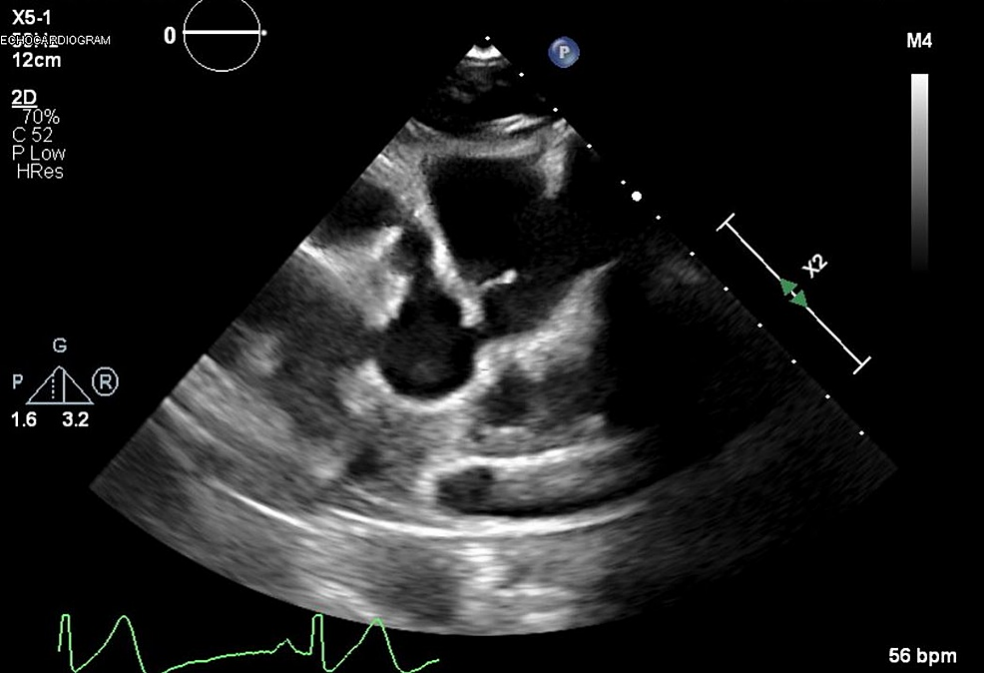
TTE-modified parasternal short-axis view demonstrating giant left and right coronary artery aneurysms TTE, Transthoracic echocardiography.
